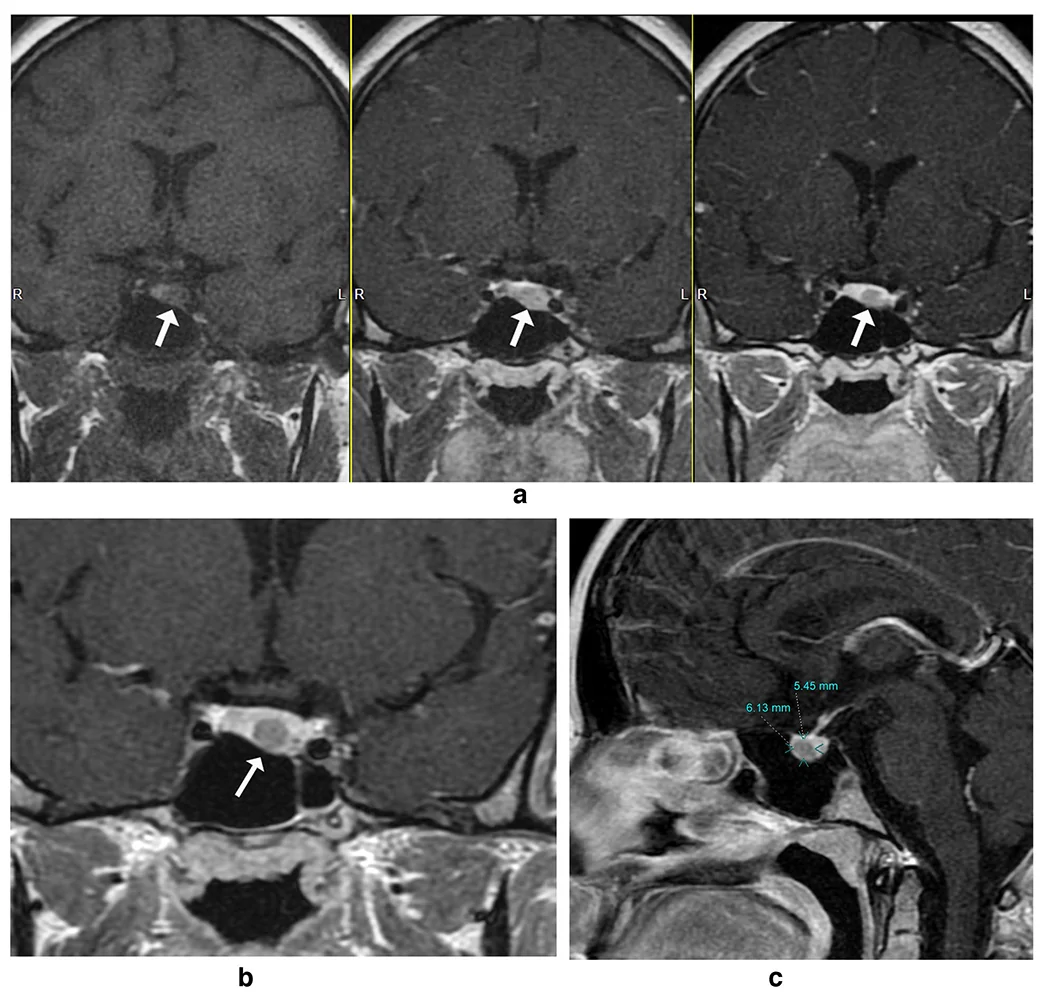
Microadenoma in a 15-year-old female with elevated prolactin levels. a Coronal dynamic post-contrast T1-weighted images through the sella show progressive enhancement of the adenohypophysis, delineating a non-enhancing round region (*arrows*), consistent with microadenoma. b Coronal and (c) sagittal delayed post-contrast images through the pituitary show the well-demarcated non-enhancing lesion (*arrow* in b) that measures 6 mm in diameter, confirming the presence of a prolactinoma
